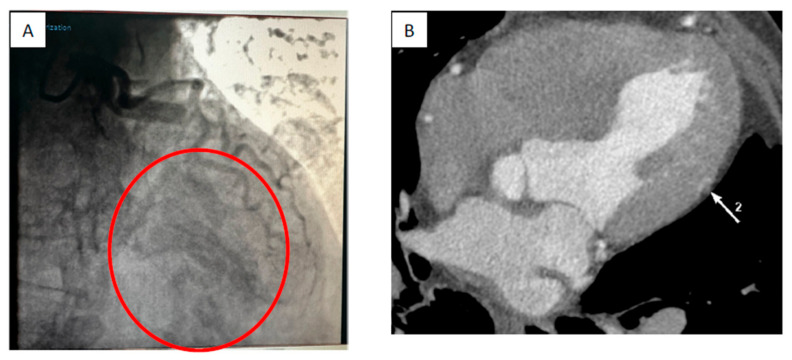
(A) Left coronary artery angiography showing drainage of contrast into the left ventricle from left anterior descending artery (circle insert); (B) contrast chest CT showing contrast into the left ventricle (arrow) from the left anterior descending artery.
